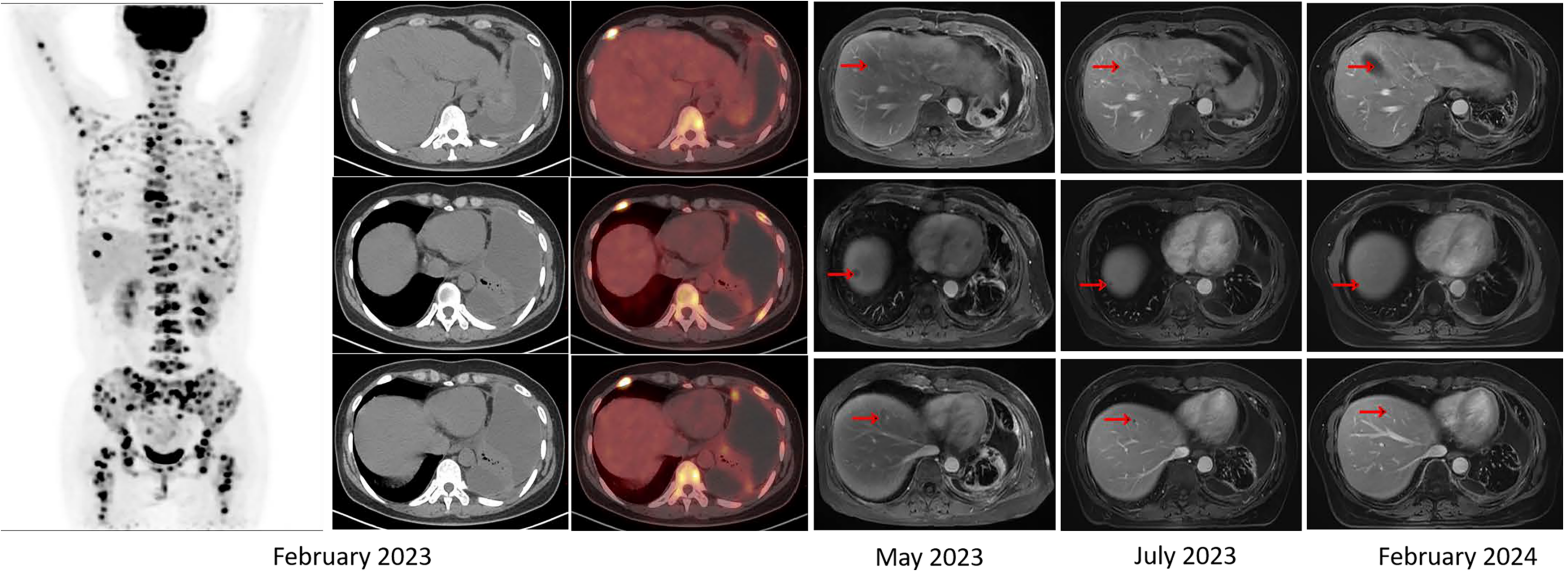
Comparison of liver imaging before and after tumor liver metastasis. In February 2023, PET/MRI results showed that the liver was in good condition and no metastatic tumors were found (in the left column). In May 2023, enhanced CT results indicated multiple liver metastases (in the third column from right to left). In July 2023, liver metastases gradually disappeared after a period of chemotherapy (in the second column from right to left). In February 2024, liver metastases completely disappeared (in the right column).
